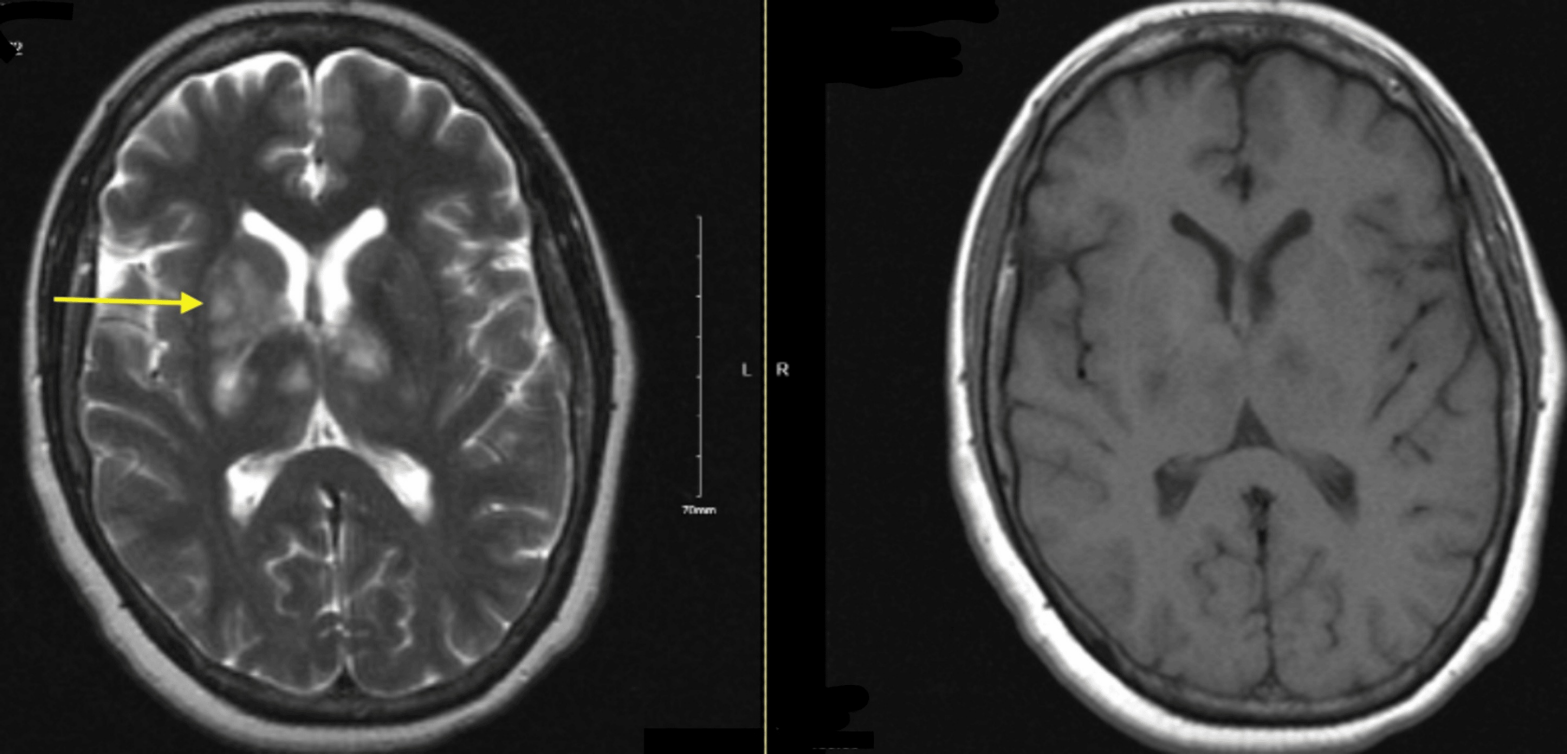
Repeat brain MRI and T1 with gadolinium. Repeat brain MRI showed mildly increased bilateral thalamic FLAIR/T2 signal and an enlarged right basal ganglia lesion (4.3 x 1.7 cm) (left image, yellow arrow). Right image shows T1 with gadolinium. FLAIR: fluid-attenuated inversion recovery.
